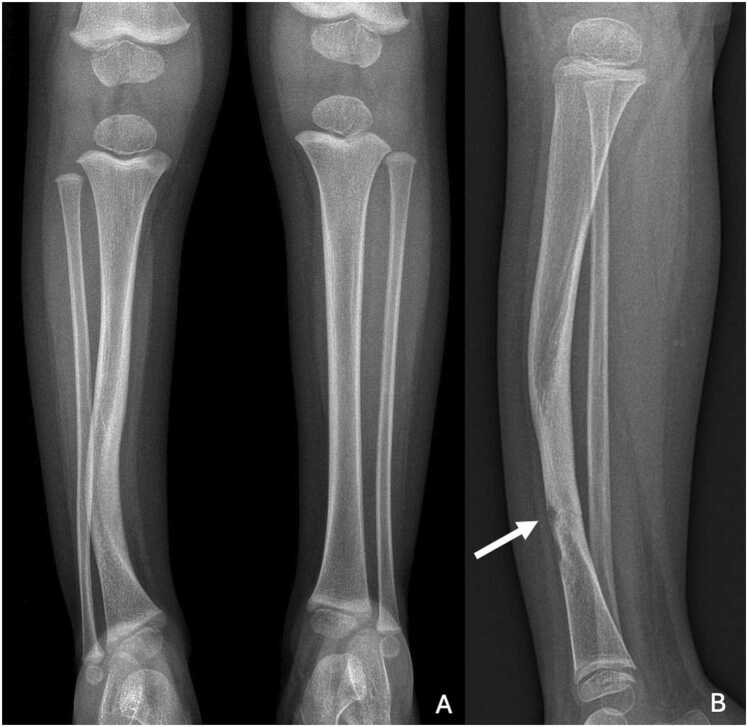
Right congenital tibial incurvation in two-year-old girl with a history of neurofibromatosis type 1, complicated by fracture. A. Face radiography with anterolateral convexity of the right tibia. B. Profile radiography performed a year later showing a fracture of the distal third of the tibia (white arrow).
